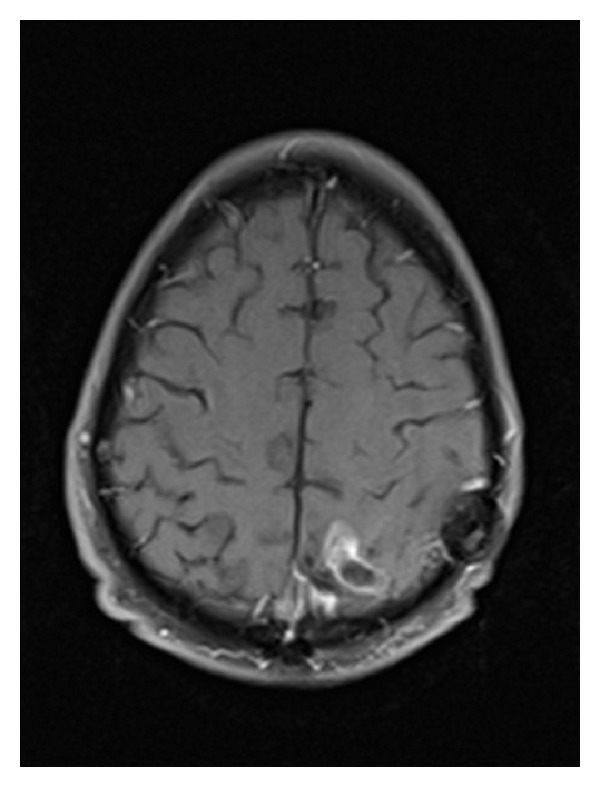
MRI scan showing increased enhancement, indicating tumor recurrence twenty-two months after GKRS salvage treatment.
